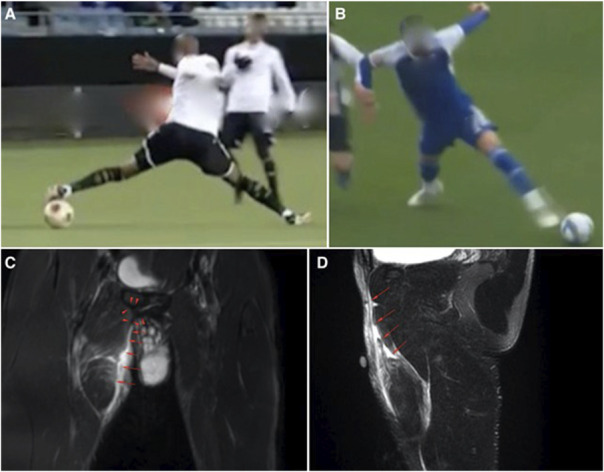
Adductor injuries: Severe adductors injuries often occur during closed kinetic chain stretching movements, such as reaching for ball with the uninjured leg (A, B). These injuries are typically characterized by hip extension, abduction, and external rotation. Examples of the possible MRI findings showing acute proximal adductor longus tear are presented (C, D). Among other possible adductor injury mechanisms are open kinetic chain stretching (pass kick, reaching with injured leg etc.) and rapid movements, such as sprinting, dribbling, and changing direction. The mechanisms are often more complicated in adductor injuries.
